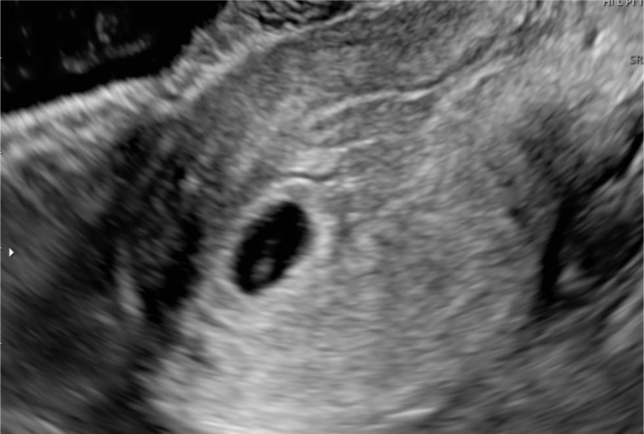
Transvaginal ultrasonography: a 12.1-mm gestational sac in the uterus.
